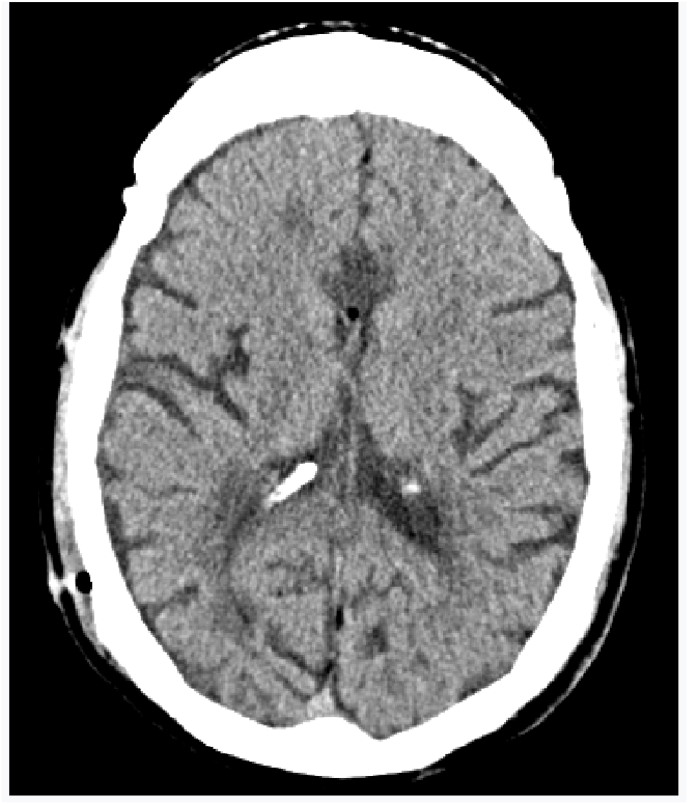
Follow op CT scan next day revealed the regression of hydrocephalus.
